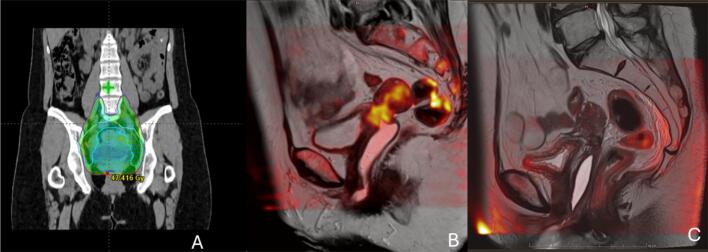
Complete response in patient 2 with salvage CRT. A. Radiation plan before CRT. B. Pre-treatment fusion MRI of the pelvis, showing the extent of the cervical relapse. C. Fusion MRI of the pelvis after CRT showing complete response of the cervical lesion.
